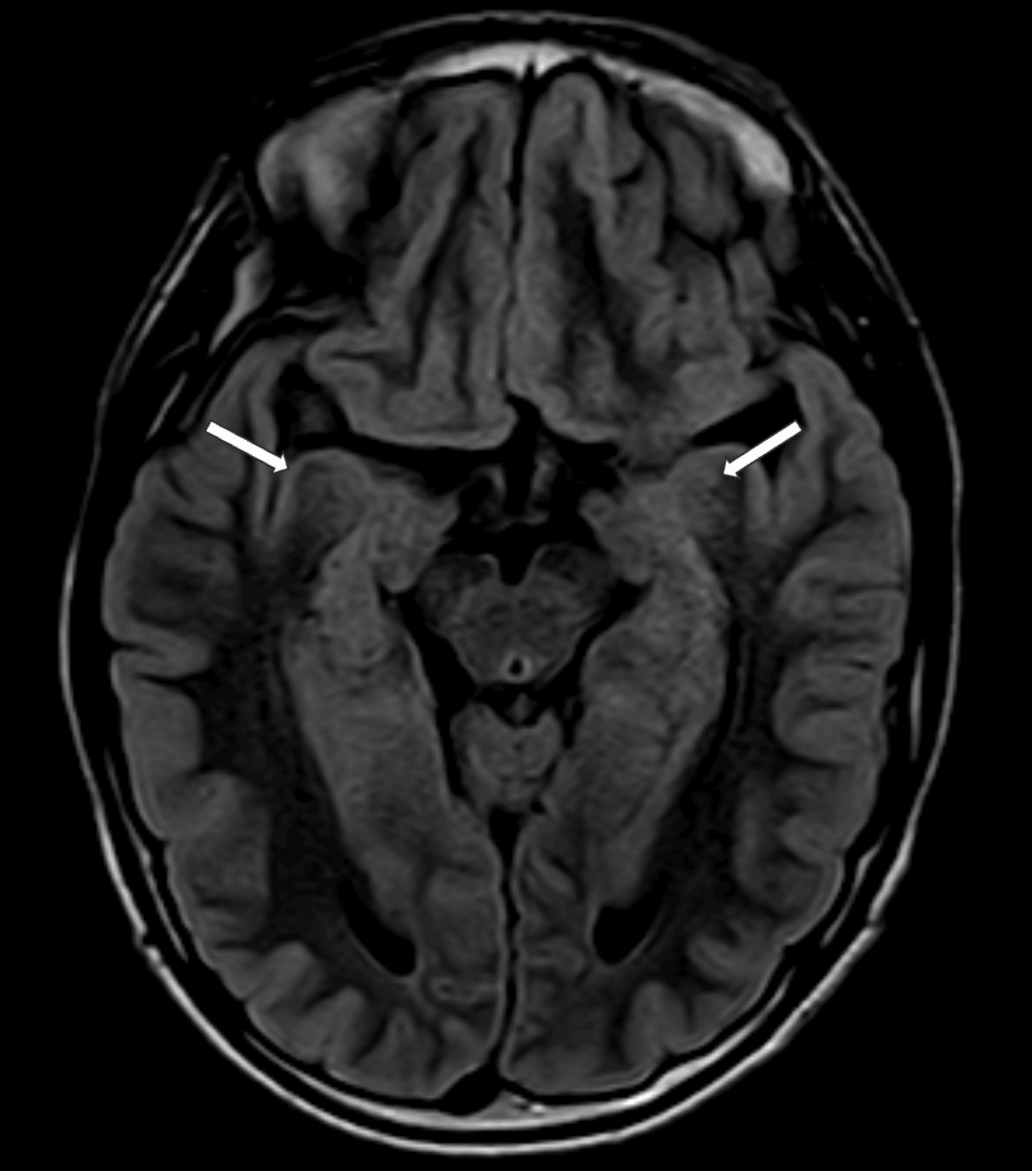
Axial-FLAIR MRI of the brain without contrast through the level of the hippocampal formations as companion to Figure 4 (white arrows) FLAIR - fluid-attenuated inversion recovery
